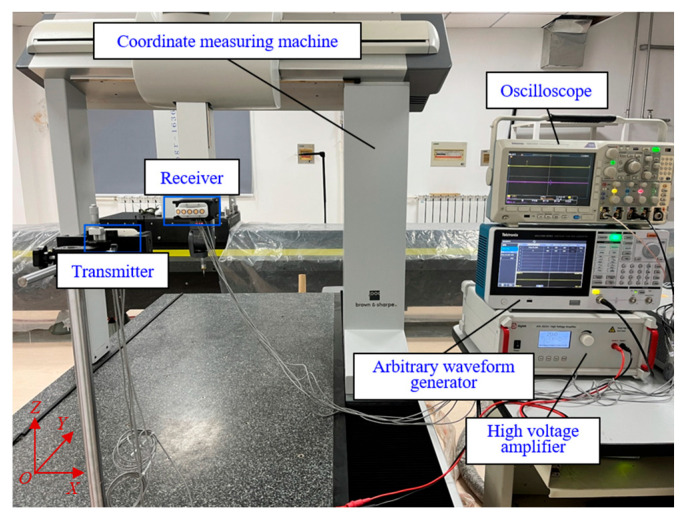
The experiment platform of the coded ultrasonic ranging system.
